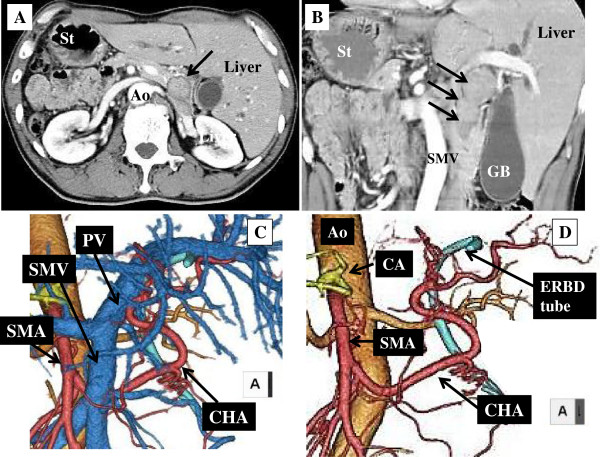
Preoperative imaging study of patient 2. (A) Contrast-enhanced computed tomography scan and (B) coronal image of multidetector-row CT show the tumor from the proximal to the distal portion of the bile duct (arrows). (C,D) Three-dimensional angiography with multidetector-row CT shows a left-to-right reversal of the vascular arrangement and that the common hepatic artery arises from the SMA and runs behind the SMV. Ao, aorta; CA, celiac artery; CHA, common hepatic artery; ERBD tube, endoscopic retrograde bile duct drainage tube; GB, gallbladder; PV, portal vein; SMA, superior mesenteric artery; SMV, superior mesenteric vein; St, stomach.
